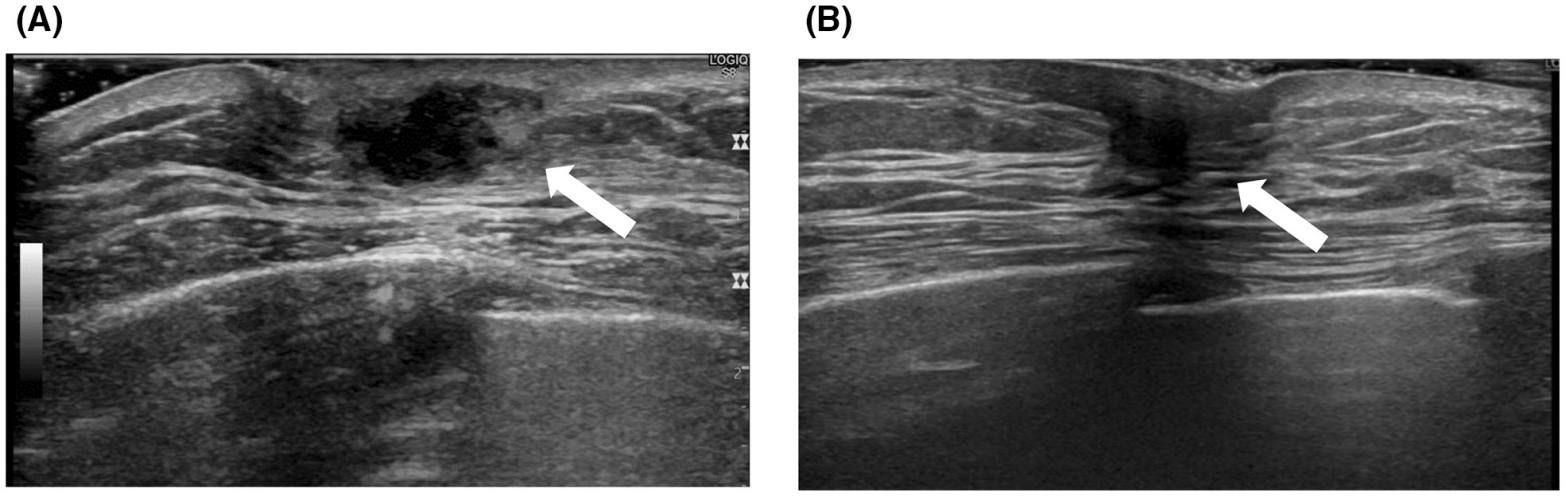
(A) Ultrasonogram at the first visit. An ill‐defined hypoechoic irregular mass with peripheral vascularity (Arrow). (B) Ultrasonogram after 3 months. Tumor size reduced to 7.9 × 5.5 × 5.2 mm (Arrow).
